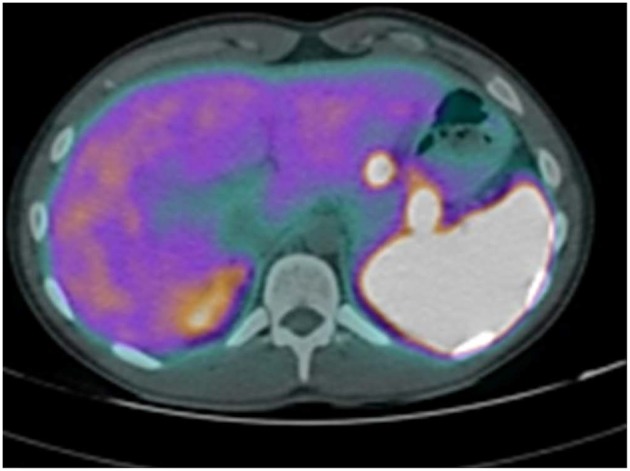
Gallium-68 labeled somatostatin receptor PET-CT showed uptake in 3 Gallium-68 labeled somatostatin receptor PET-CT showed an uptake in 3 nodules in the pancreas.
